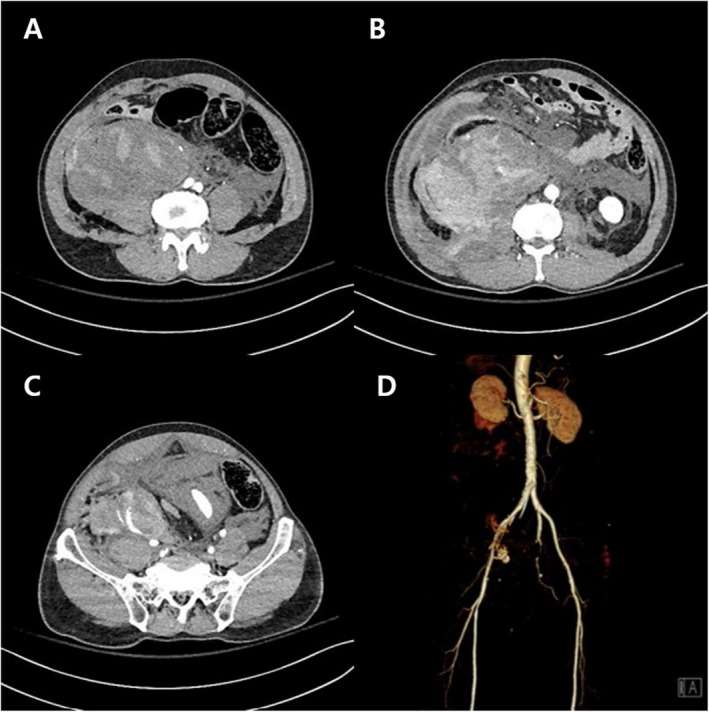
Computed tomography and CT angiography demonstrating retroperitoneal hemorrhage due to proximal common femoral artery rupture. (A–C) Axial computed tomography images showing a massive retroperitoneal hematoma extending along the iliopsoas muscle. (D) Three‐dimensional CT angiography demonstrating active contrast extravasation from the proximal common femoral artery, remote from the femoral puncture tract.
